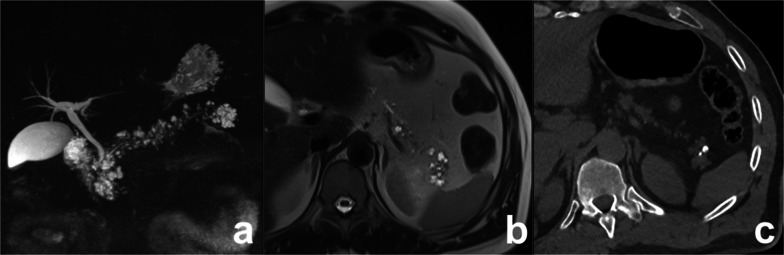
Acinar cell cystadenoma. At MRCP (a), there are several small cysts thought the pancreas. On T2w (b), some small hypointense foci are appreciable in the tail of the pancreas which at CT show up to be calcifications (c). At biopsy, an acinar cell cystoadenoma was found
